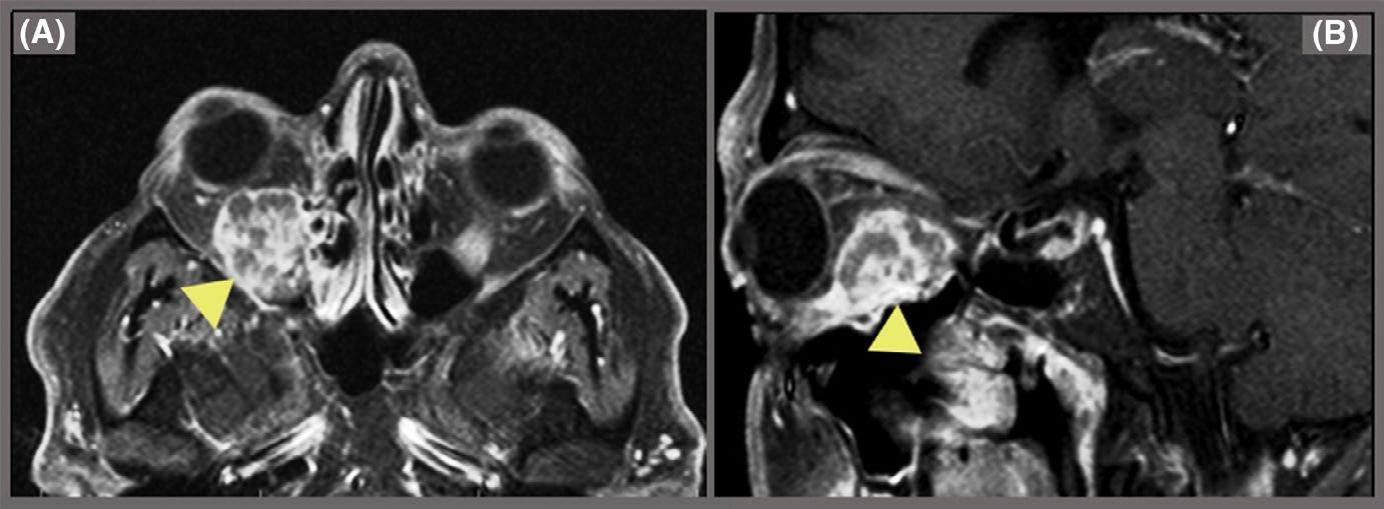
T1‐weighted MRI images of the right orbit show an intracanal space‐occupying lesion, which has caused significant proptosis (A: horizontal view, B: sagittal view)
